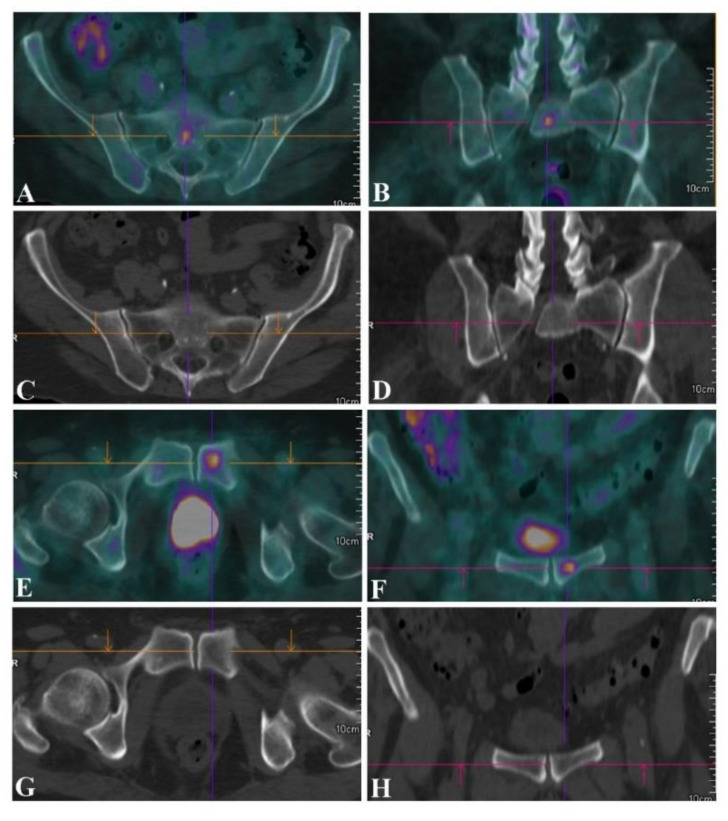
(A,B; SUV_max_ = 7.1) ^18^F-FDG PET/CT for initial staging in a patient with a rhabdomyosarcoma of the right thigh showing unexpected small FDG-avid bone metastases at the level of the sacrum (A,B; SUV_max_ = 7.1) and of the left hemipubis (E,F; SUV_max_ = 7.9), which were not associated with any osteostructural alteration at the (C,D,G,H) co-registered CT image. (A,E) Axial and (B,F) coronal ^18^F-FDG PET/CT fused images of the sacrum and of the hip, along with the (C,D,G,H) corresponding CT images.
